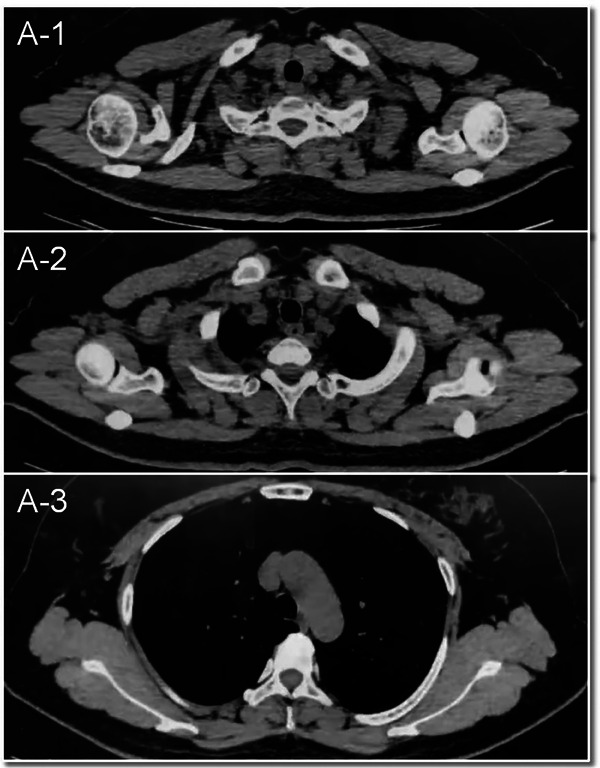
Preoperative plain CT scan.
